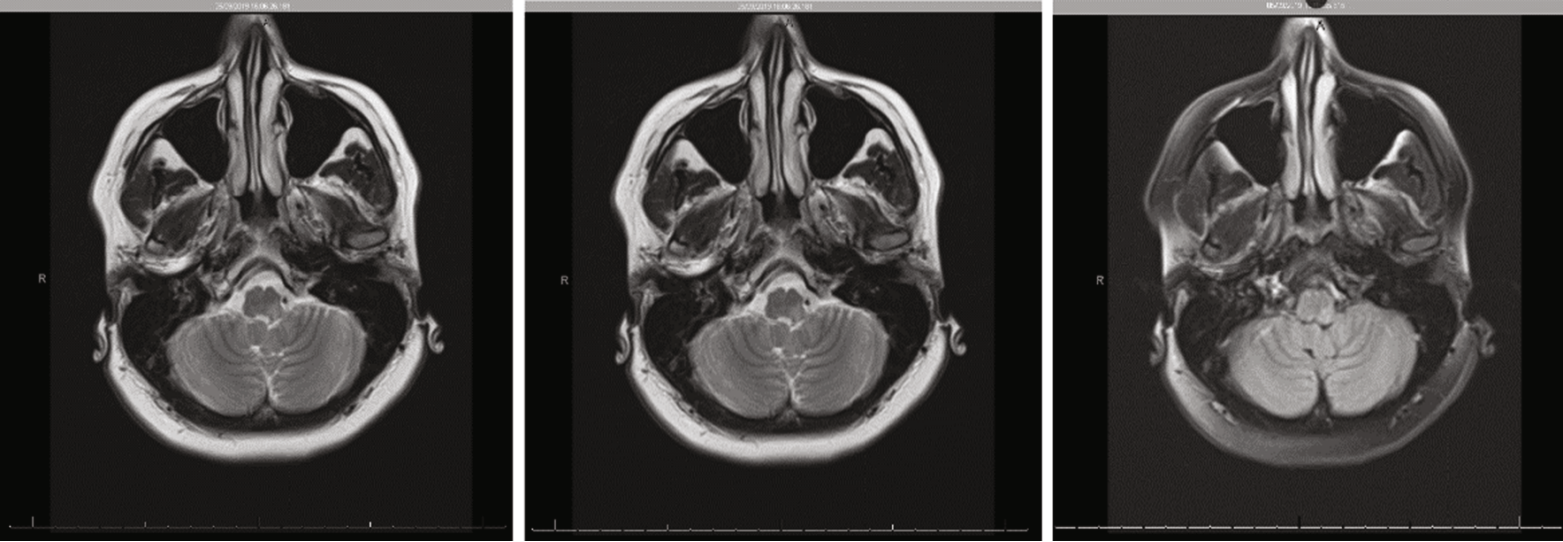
MRI of the brain with and without contrast showing focal restricted diffusion within the left posterolateral aspect of the medulla with associated focal heterogeneous T2 hyperintense signal, indicative of an evolving focal subacute cerebrovascular accident. Alternatively, this finding may represent an acute-subacute focus of demyelination in the proper clinical setting.
